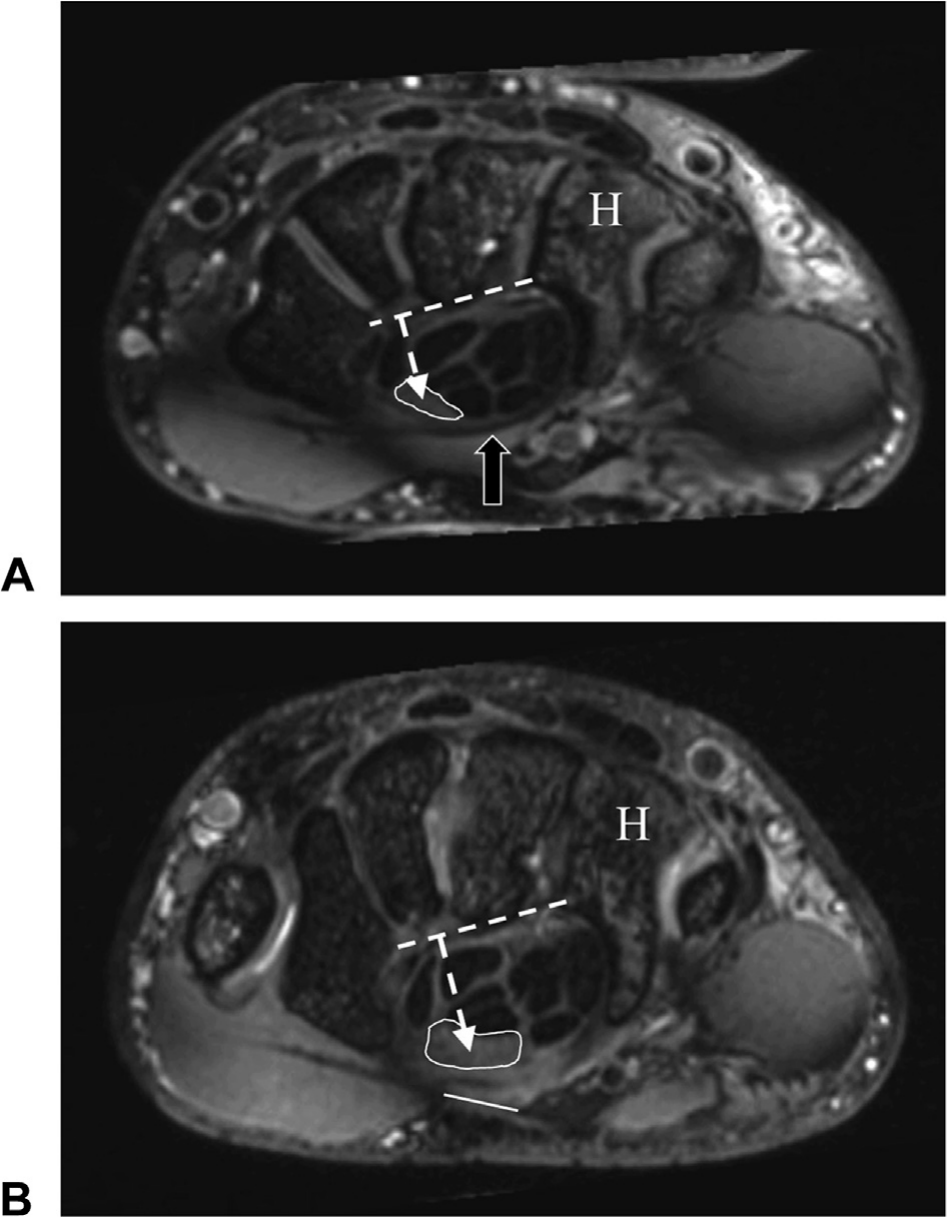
A Preoperative T2 weighted axial MRI at the hamate (H) level shows the cross-sectional area of median nerve (white outline) with the transverse carpal ligament intact (arrow). B 3-months postoperative T2 weighted axial image at the hamate level shows an increase in the cross-sectional area of median nerve (white outline) and a gap in the transected transverse carpal ligament (white line). Dashed line and arrow in images A and B show a palmar shift of median nerve reported as the difference in postoperative distance measured from the palmer aspect of the carpals (dashed line) and the center of the median nerve (dashed arrow).
